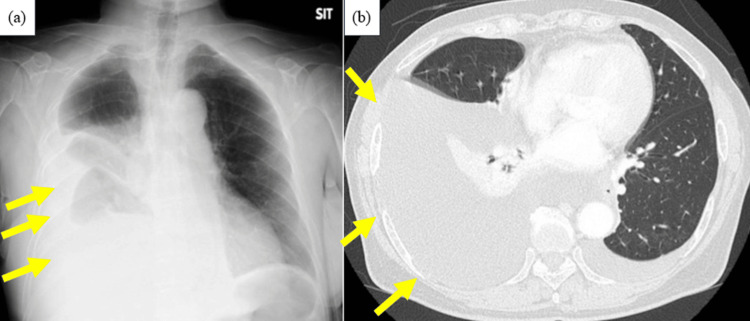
Radiography and computed tomography at emergency hospitalization (a) Radiograph and (b) computed tomography showing a right-sided predominant pleural effusion (indicated by arrows).
